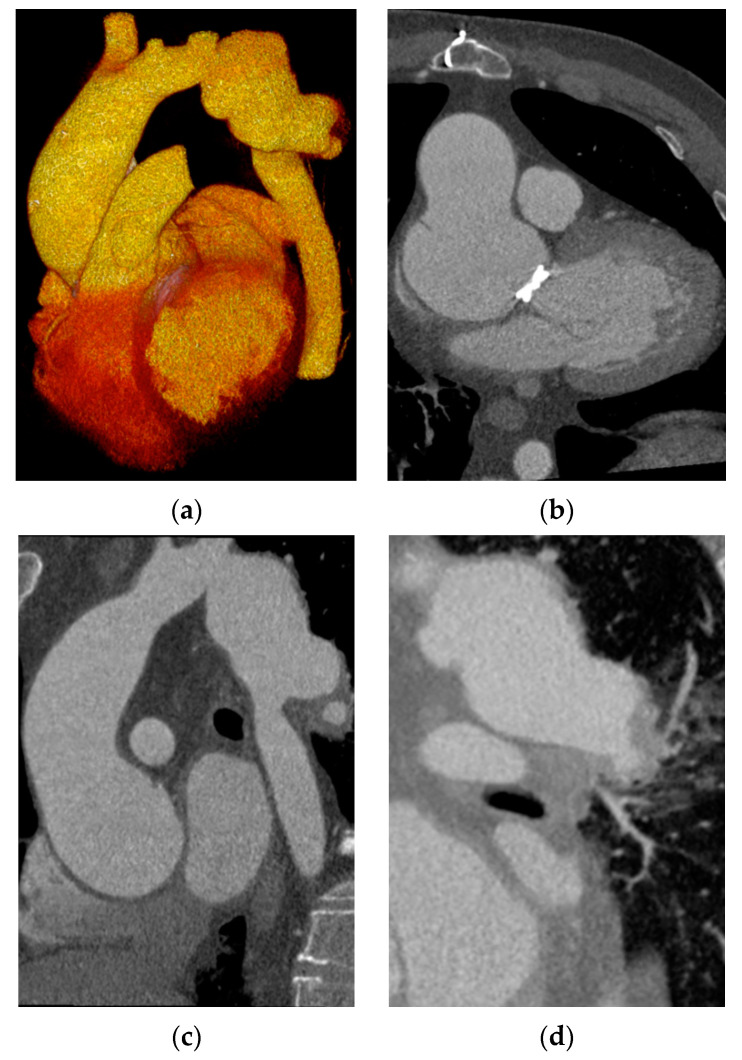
Cardiac CT angiography: (a) 3D VRT (volume rendering technique) reconstruction of the aorta showing the PSA; (b) closed mechanical valve and dilated ascending aorta with chronic limited dissection; (c) pseudoaneurysm of the descending thoracic aorta immediately after the ostium of the left subclavian artery; (d) thickened left lower lobe apical segmental bronchus in contact with the PSA and adjacent alveolar hemorrhage.
